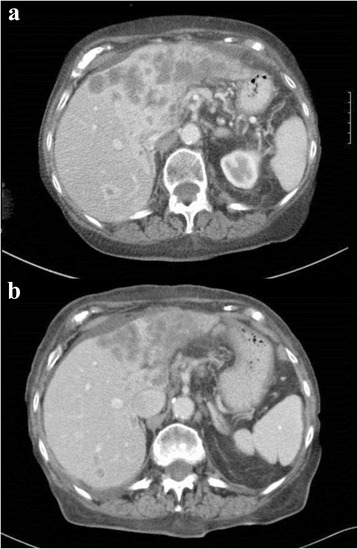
Pre-treatment and post-treatment imaging. a Pre-treatment CT scan of liver. b Post-treatment CT scan of liver
